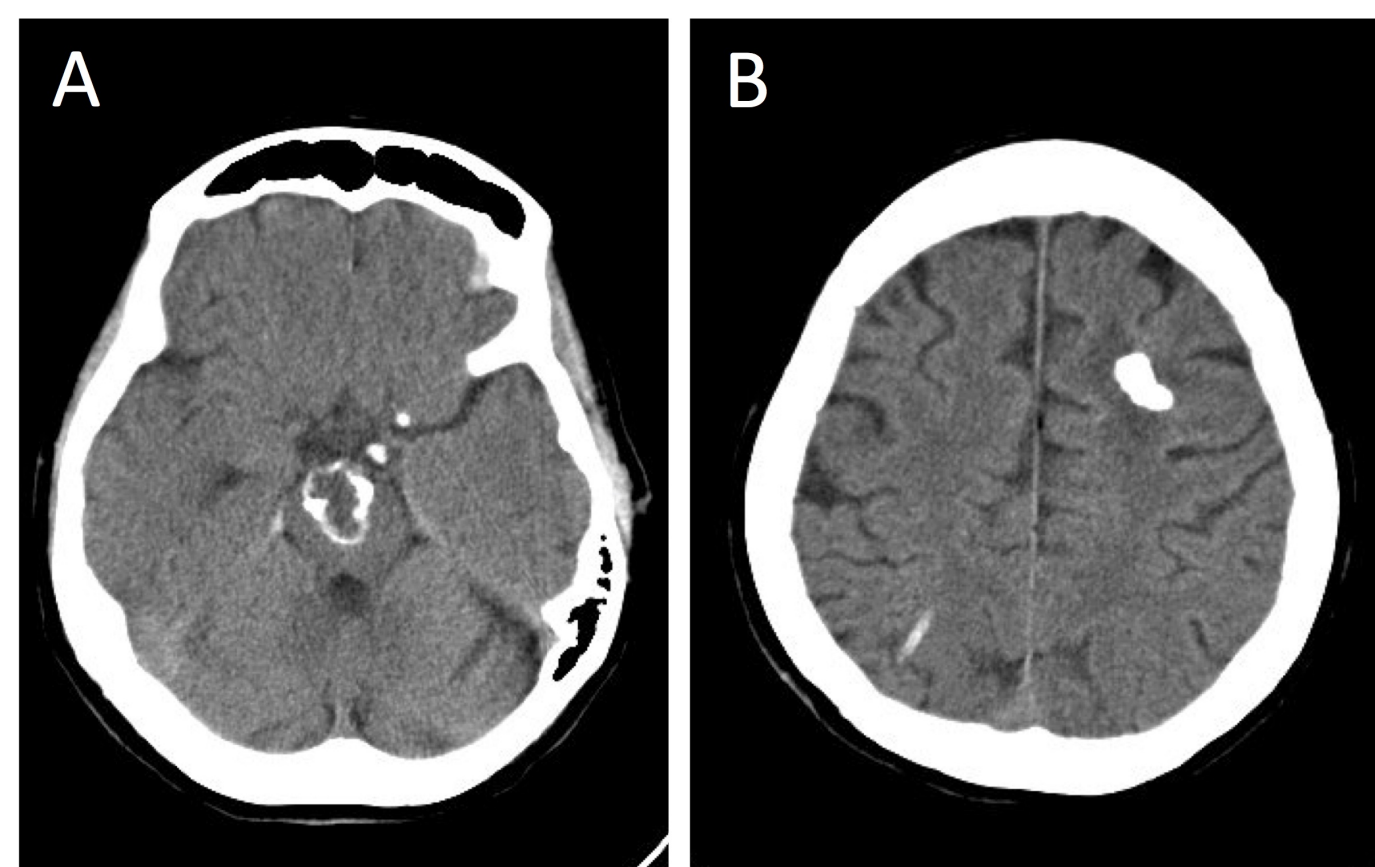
Computed tomography showing multiple calcified lesions Computed tomography (CT) images. Axial brain window images depicting hyperdense, calcified lesions in the brainstem (A) and left frontal lobe (B).
